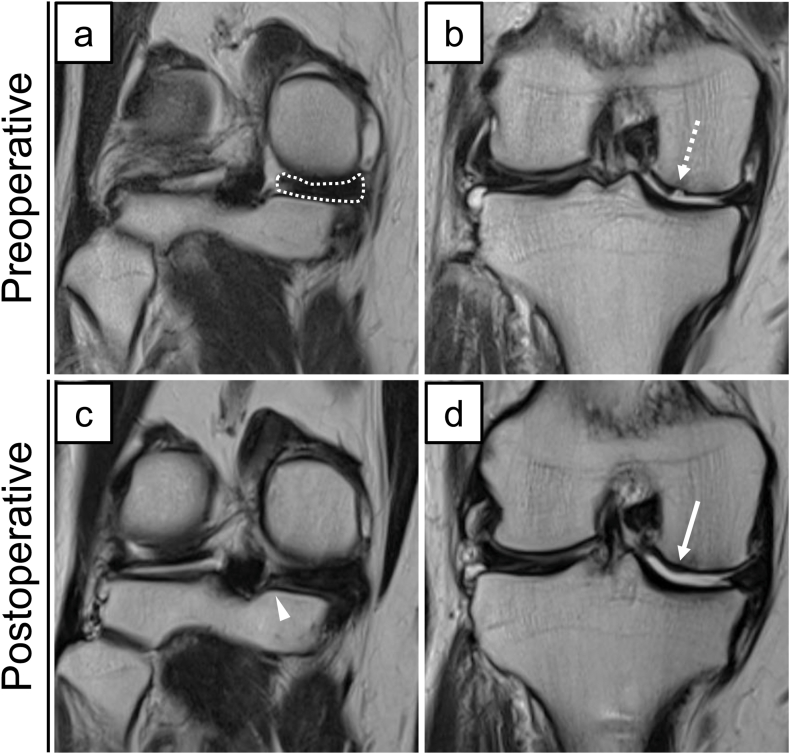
Representative magnetic resonance images of the right knee undergoing pullout repair for medial meniscus (MM) posterior root tear (PRT) combined with microfracture. a) Preoperative coronal image showing giraffe neck sign (white dotted area), indicative of MMPRT. b) Preoperative coronal image showing a cartilage lesion on the medial femoral condyle (MFC). c) Postoperative coronal image showing restored continuity of the MM posterior root. d) Postoperative coronal image showing improvement in the cartilage lesion on the MFC.
